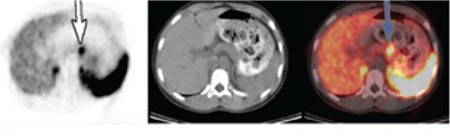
Positron emission tomography - computed tomography imaging showing increased uptake in the right adrenal gland and 11x10 mm nodular lesion in corpus of the pancreas
